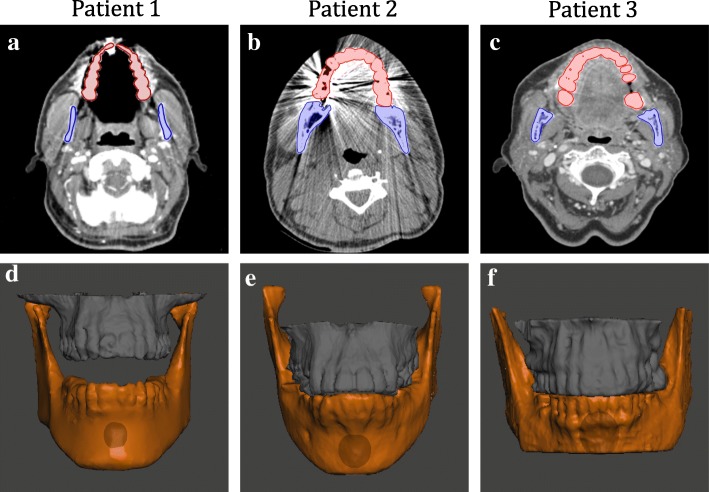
Manual segmentation of maxilla and mandible on pre-treatment CT scans of 3 patients (a, b, c) and 3D reconstruction of the segmented bone and teeth (d, e, f)
